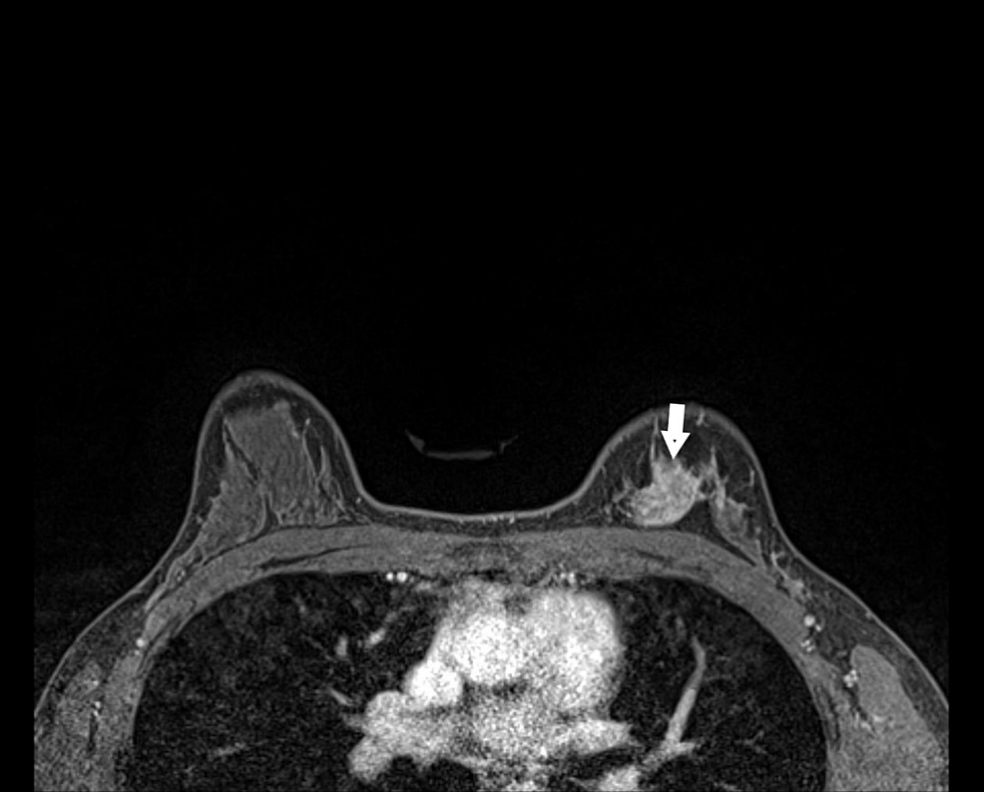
Single axial T1W post-contrast MRI image demonstrating enhancement in the upper inner quadrant of the left breast (white arrow).
